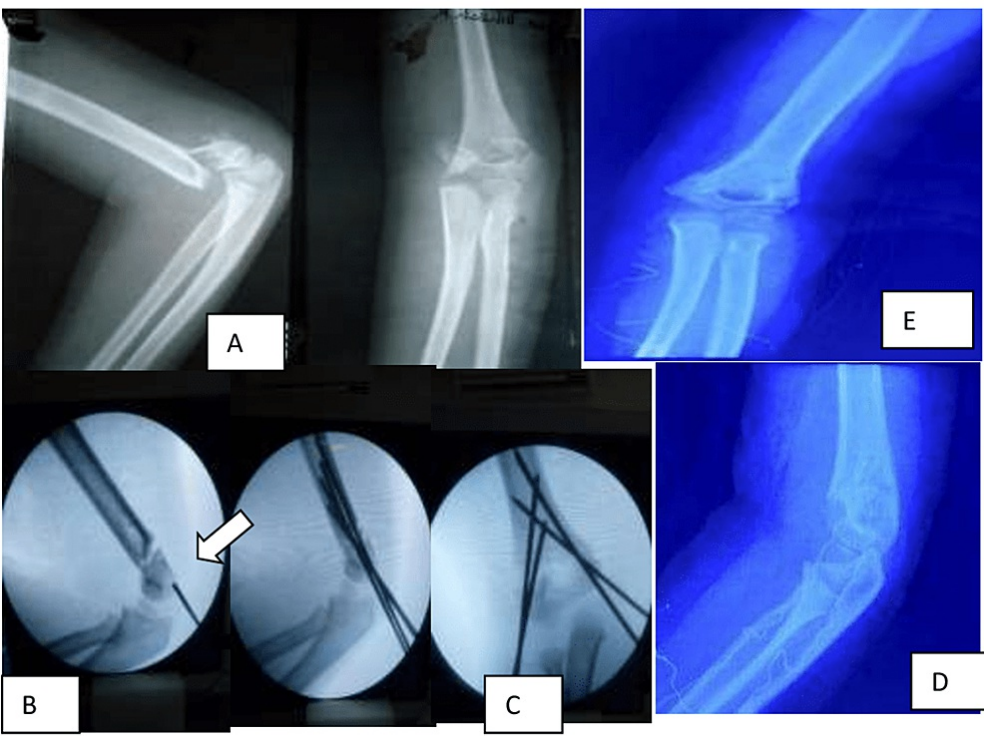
Preoperative X-ray anteroposterior and lateral views showing a Gartland type III supracondylar fracture (A) Preoperative X-ray, (B) Putting the medial wire first as shown by arrow, (C) postoperative x-ray, (D&E) At three weeks following wire removal
